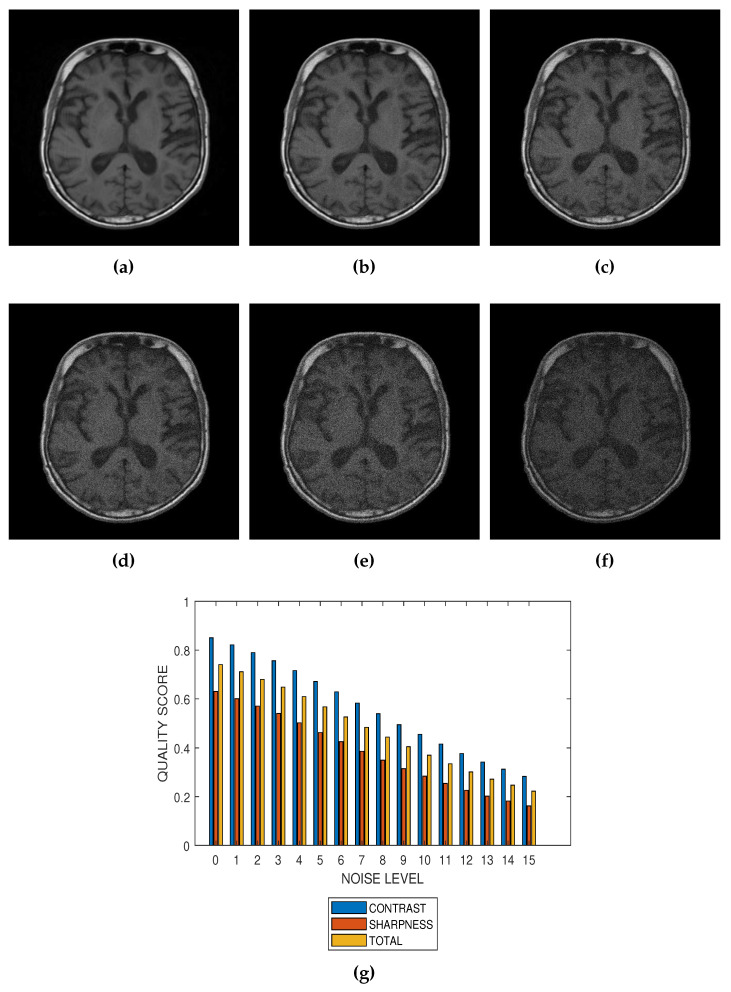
(a) A conventional T1 weighted MRI slice from BrainCare Oy., and its degraded versions at Rician noise levels (b) 3 percent, (c) 6 percent, (d) 9 percent, (e) 12 percent and (f) 15 percent. (g) Variation of contrast, sharpness and total quality scores for Rician noise levels that vary from 0 percent to 15 percent.
